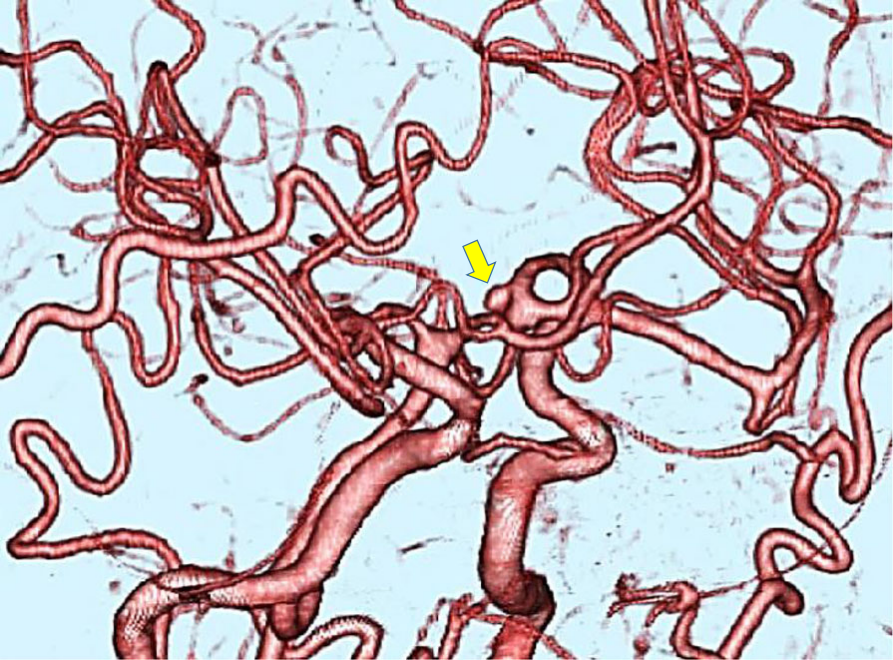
CT angiography (CTA) showing an aneurysm of which size was about 3.0 mm between A1 and A2 of the left anterior cerebral artery. The aneurysm is indicated by an *arrow*
